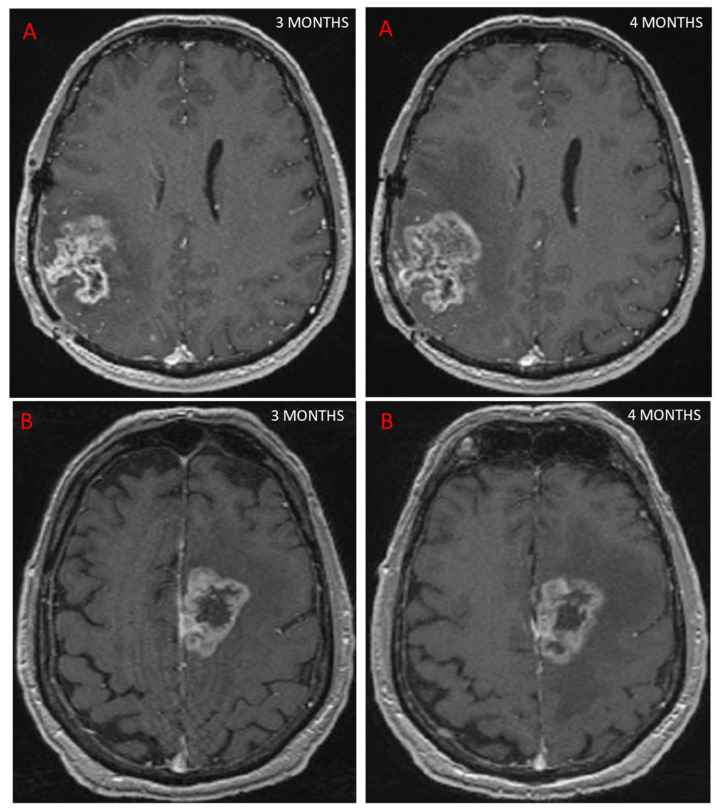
This figure shows two patients treated for GBM with concurrent RT and chemotherapy. At 3 months, MRI showed an increase in contrast-enhancing lesion on axial T1 sequence after injection of a contrast agent, suggestive of progression in both cases. Further MRI scans performed 1 month later (4 months post-RT) showed that one patient had TP, with an increase in contrast (A), while the other patient had PsP, as the contrast remained stable (B).
